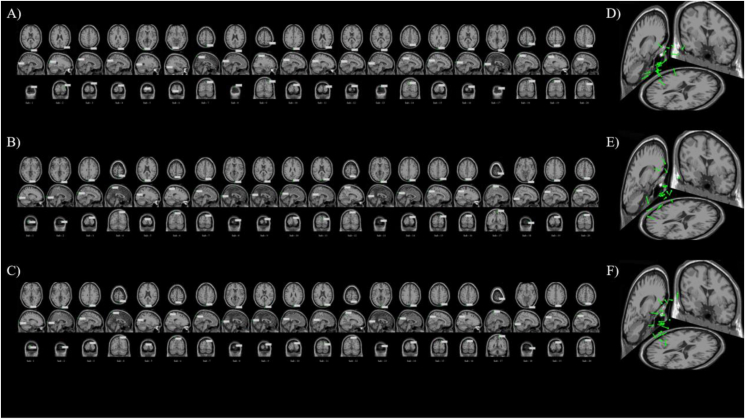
Space location reconstruction. Figure 16-A), B), C) The left inset function anatomy image from 20 subjects, on the MRI 3D-plot (right side view, rear view, and top view). Dipole location under the guided of machine learning decoding result. D E F The right inset function anatomy image from 20 subjects, reconstructing the machine learning decoding guided dipole space location.
